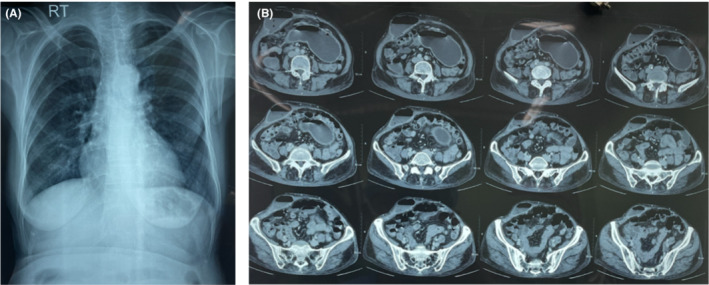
(A) Chest X‐ray without evidence of pneumoperitoneum; (B) CT scan showing a subcutaneous (54 mm × 28 mm) right anterior abdominal wall collection with air fluid level in continuity with a collection within the abdominal cavity (87 mm × 12 mm), further continuous with another collection near the left border of the stomach (90 mm × 23 mm) with suspicious breach of the pylorus of the stomach.
